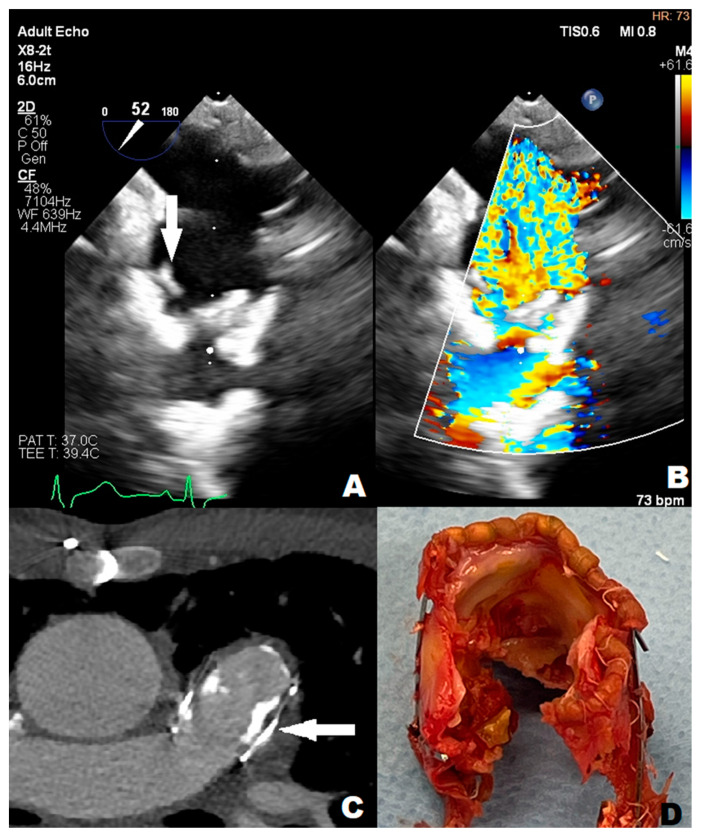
(A) arrow point at slightly-built, ballotable vegetation well-visible in TOE (B) BioPulmonic regurgitation wave in TOE; (C) arrow point at BioPulmonic metal frame visible in angio-CT; (D) the BioPulmonic PV after cardiac surgery; TOE—transesophageal echocardiography; angio-CT—angio computed tomography.
